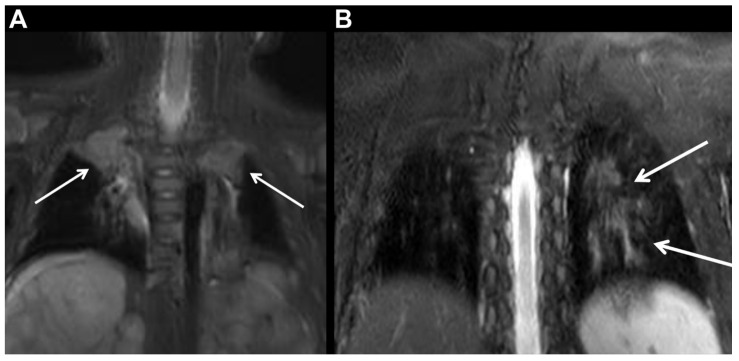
(A) A five-month-old male patient diagnosed with neuroblastoma and multiple hepatic metastases was sedated with intravenous propofol for whole-body magnetic resonance imaging. The initial image (coronal short tau inversion recovery image of thoracic level) shows segmental atelectasis (Grade 4; arrow) in both upper lobes. The estimated volume of atelectasis per the total lung volume was 13.6%. (B) A six-year-old male patient diagnosed with neurofibromatosis Type I was sedated with intravenous dexmedetomidine. The initial image (coronal short tau inversion recovery image of thoracic level) shows linear atelectasis along bronchovascular bundles (Grade 2; arrow) in the left lower lobe. The estimated volume of atelectasis per the total lung volume was 1.08%.
